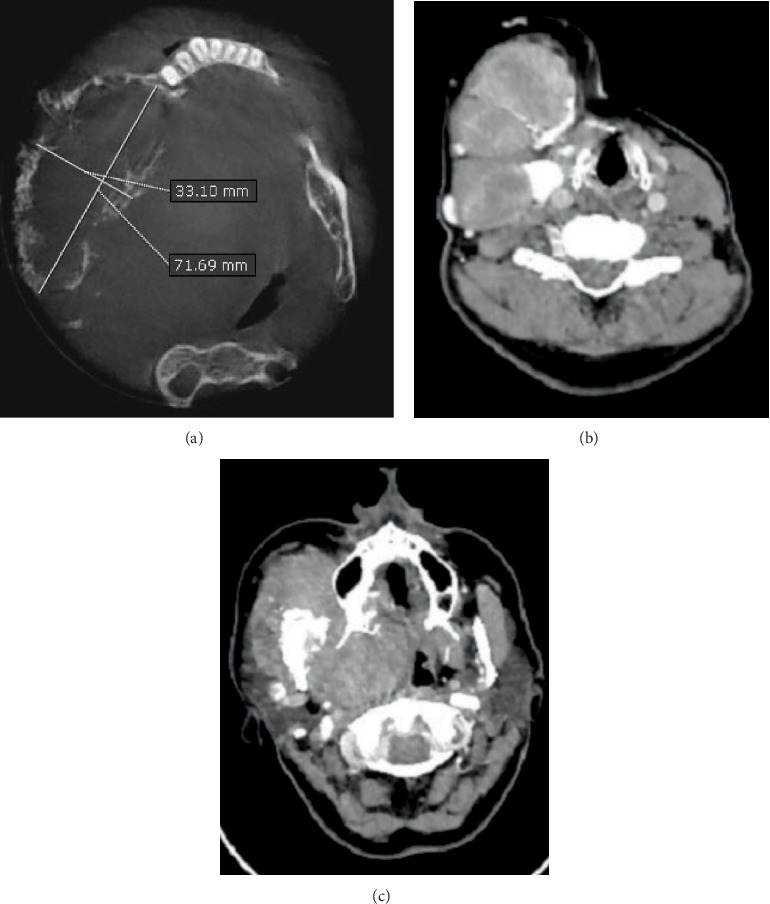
(a) Computed tomography axial plane generated by OnDemand3D App software (Cybermed Inc., Seoul, Korea) showing the anteroposterior dimension of the lesion and the buccolingual dimension. Raw computed tomography axial planes showing (b) three lobulated masses and (c) the invasion of the buccal space on the right side.
